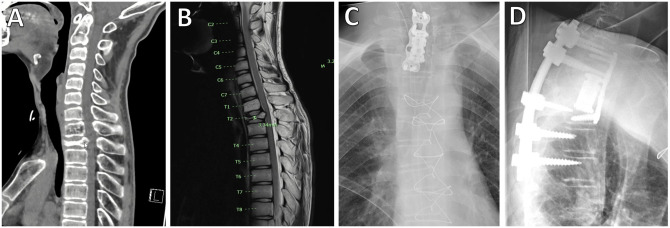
Pre-Operative and Post-Operative Imaging. (A) Pre-operative CT. (B) Pre-operative MRI. (C) Radiographic imaging following anterior plate fixation. (D) Radiographic imaging following posterior plate fixation.
